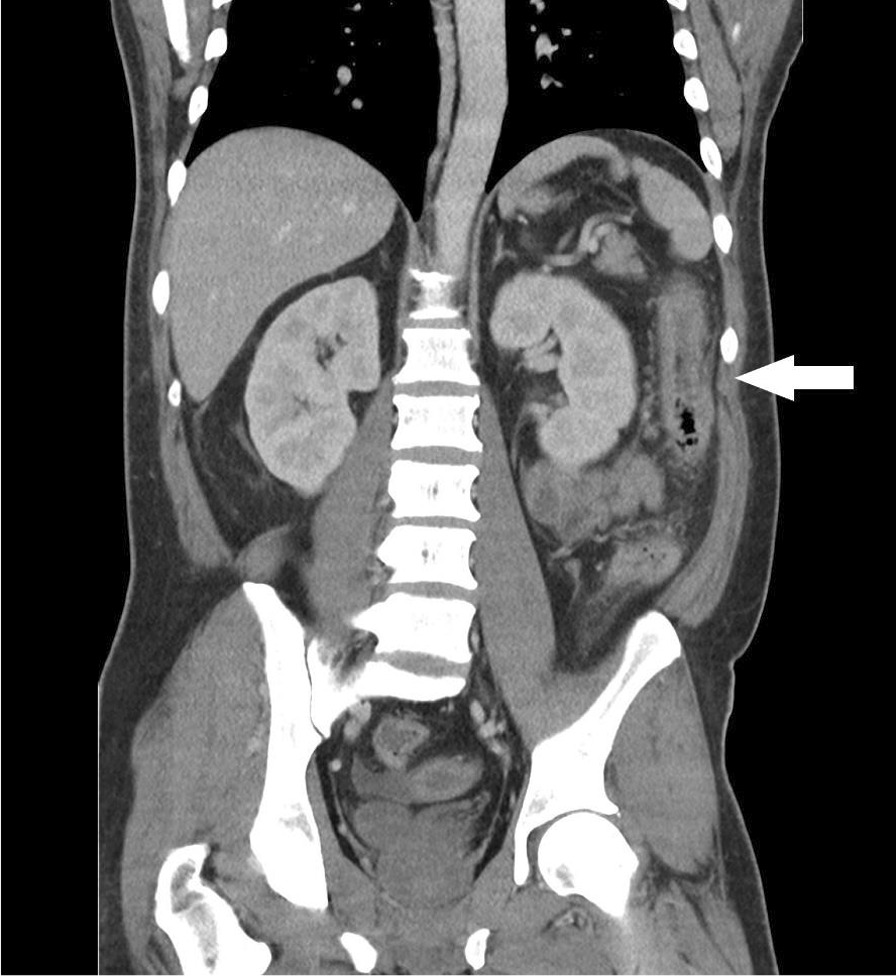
Coronal contrast-enhanced CT image shows marked circumferential wall thickening of the descending and sigmoid colon with luminal narrowing, associated mucosal hyperenhancement, as well as adjacent inflammatory stranding and edema (see arrowhead)
